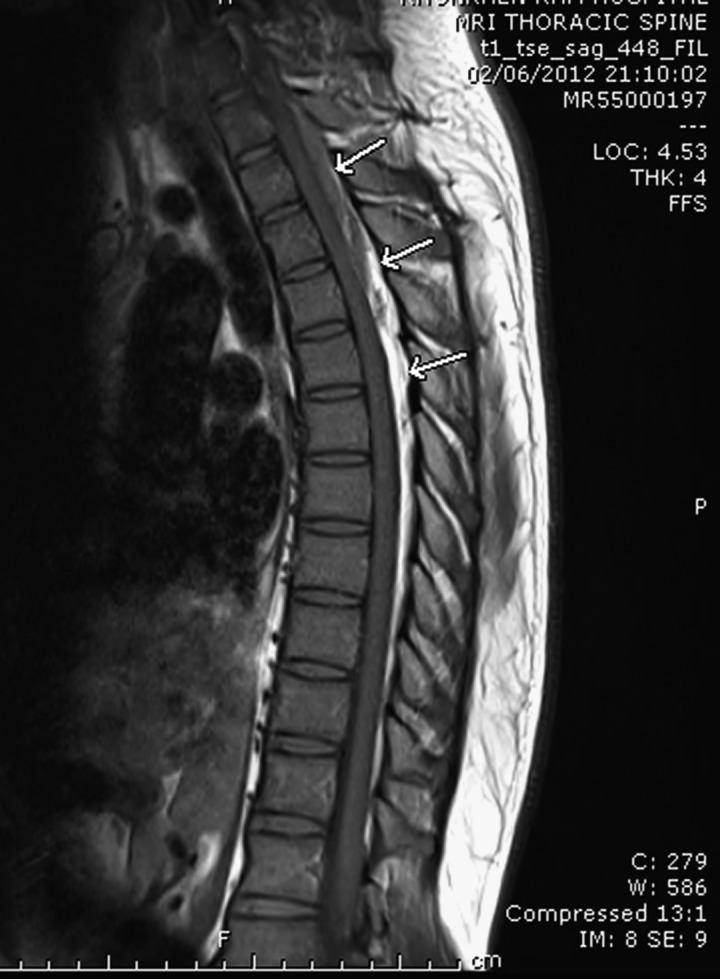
MRI of thoracic spine showed an isointensity lesion on the T1 weighted image at the posterior aspect of the thoracic thecal sac.
